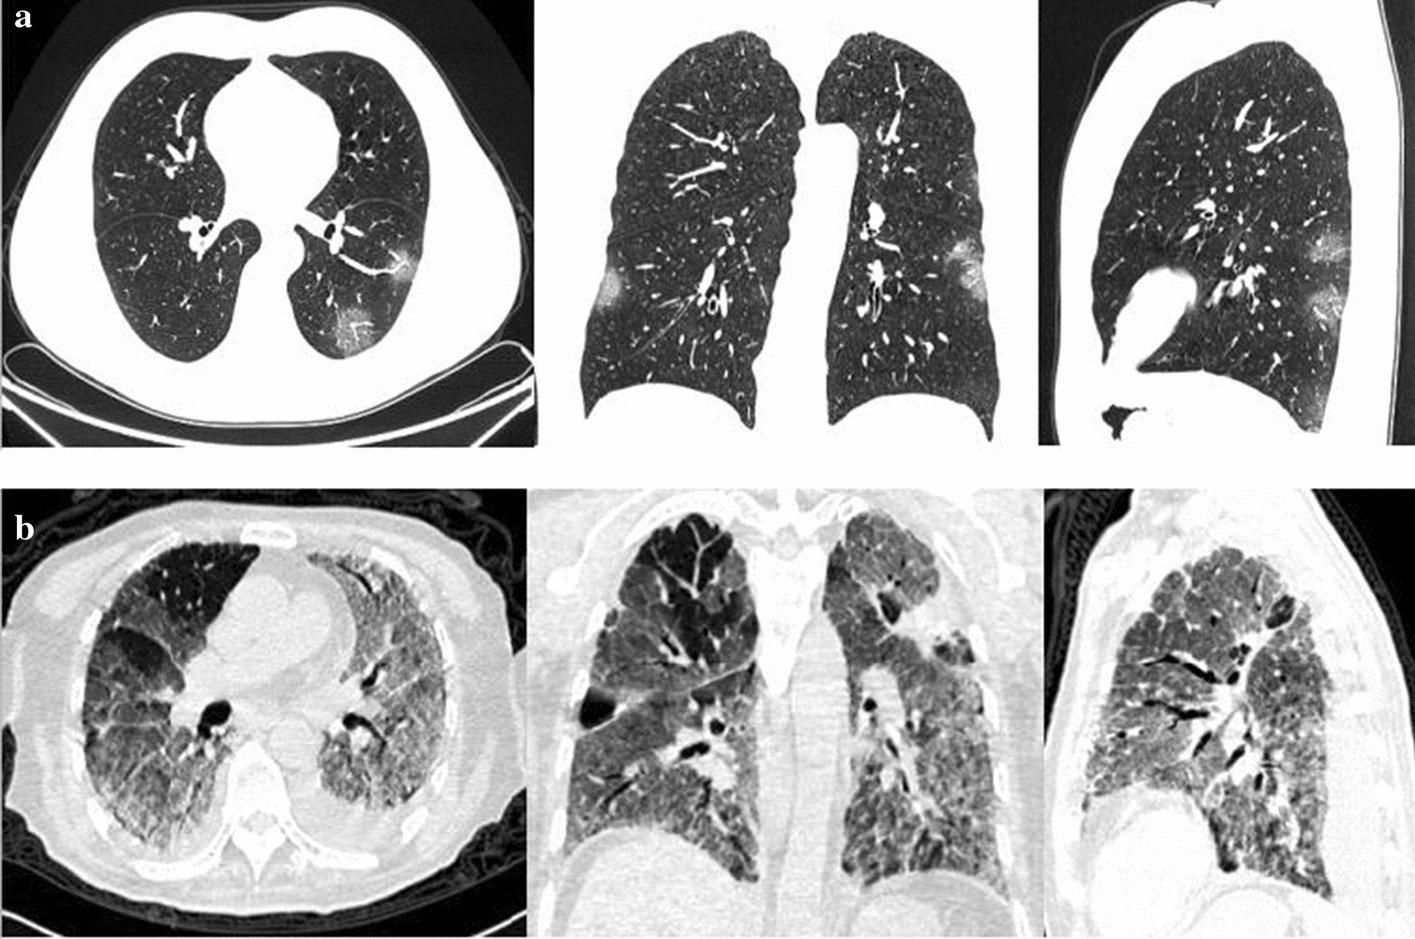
Typical examples for severe and non-severe CT chest slides in axial, sagittal and coronal views: a non-severe cases; b severe cases
